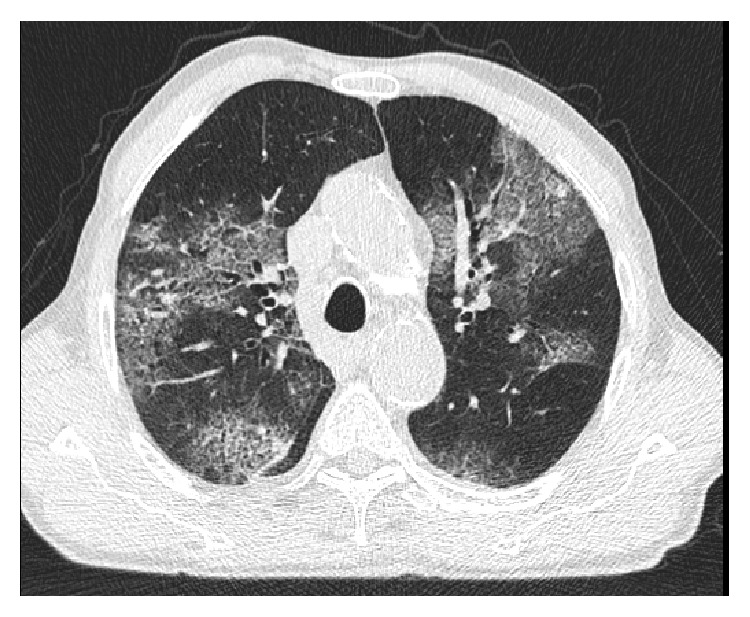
Chest CT scan done during the first admission showing bilateral patchy asymmetric interstitial lung disease suspicious of allergic alveolitis or eosinophilic pneumonitis.
